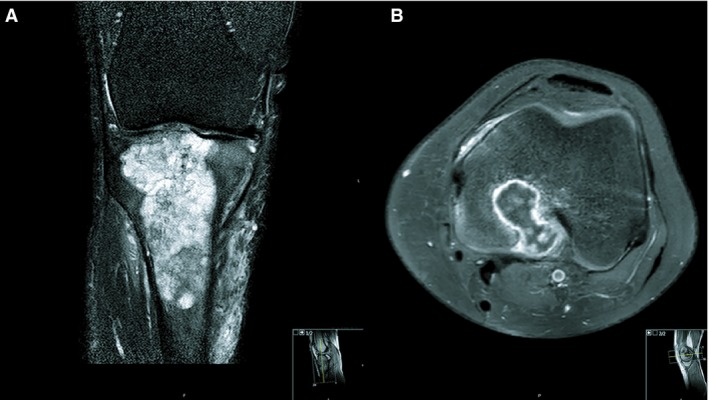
A, Magnetic resonance imaging of case 1. A coronal T2 SPIR sequence of the right knee shows a multiloculated lesion in the proximal tibia epimetaphysis. The lesion contains T2 hyperintense cartilage nodules, which are typical for chondroid tumours. The extension into the epiphysis (in this case up to the subchondral bone plate) is not typical for a chondroid tumour, which is usually contained in the metaphysis. Small internal foci of low signal intensity are noted, and are in keeping with chondroid matrix calcifications. Perilesional bone marrow oedema and soft tissue oedema are present. B, Magnetic resonance imaging of case 2. Axial T1 SPAIR after administration of gadolinium contrast in the left distal femur shows a typical septonodular or ‘rings‐and‐arcs’ enhancement in this cartilaginous tumour. The lesion extends into the epiphysis and through the posterior cortex into the soft tissues. Perilesional bone marrow oedema is present.
